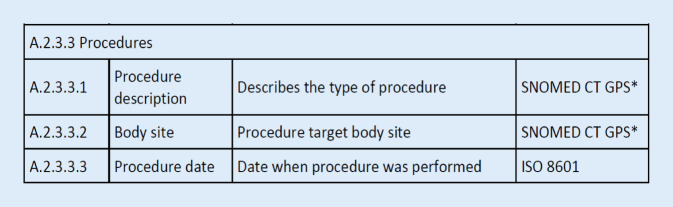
Unter anderem deshalb findet sich im MIO „Patientenkurzakte“, also dem MIO, das die relevantesten Daten einer Person zusammenfasst, auch nicht nur das Kodiersystem OPS für Prozeduren, sondern auch das Kodiersystem SNOMED CT.
